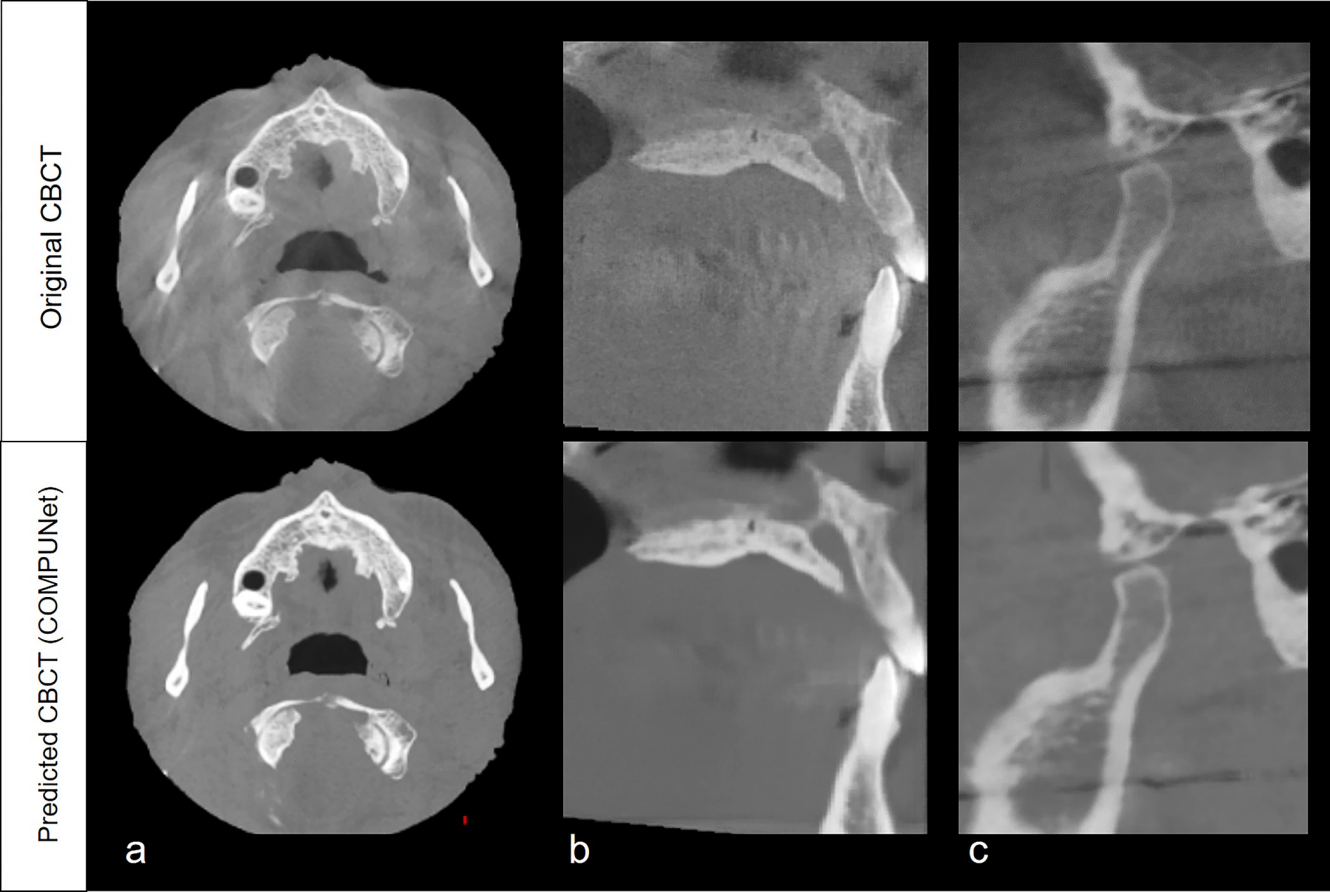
Original CBCT with an overall image grade of poor and the corresponding predicted CBCT image. (a) The maxilla in an axial view (b), the anterior teeth region in a sagittal view, and (c) the temporomandibular region in a parasagittal view show reduced artifacts and noise in the COMPUNet CBCT compared to the original CBCT images.
